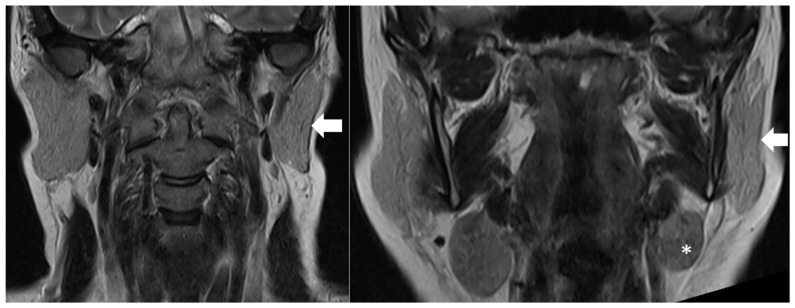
Volume loss of the left parotid gland (white arrow) and left submandibular gland (white asterisk), demonstrated on coronal T2-weighted brain MRI.
